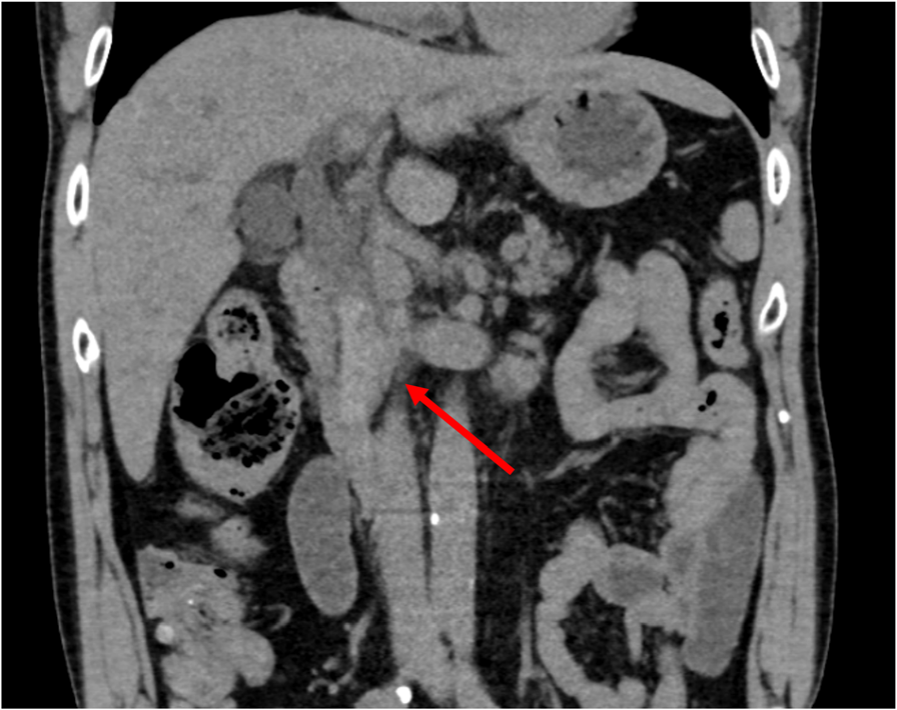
Plain CT scan image on admission. Coronal section of plain CT showing the high-density area in the CBD (arrow) and the dilation of the CBD and intrahepatic bile duct
